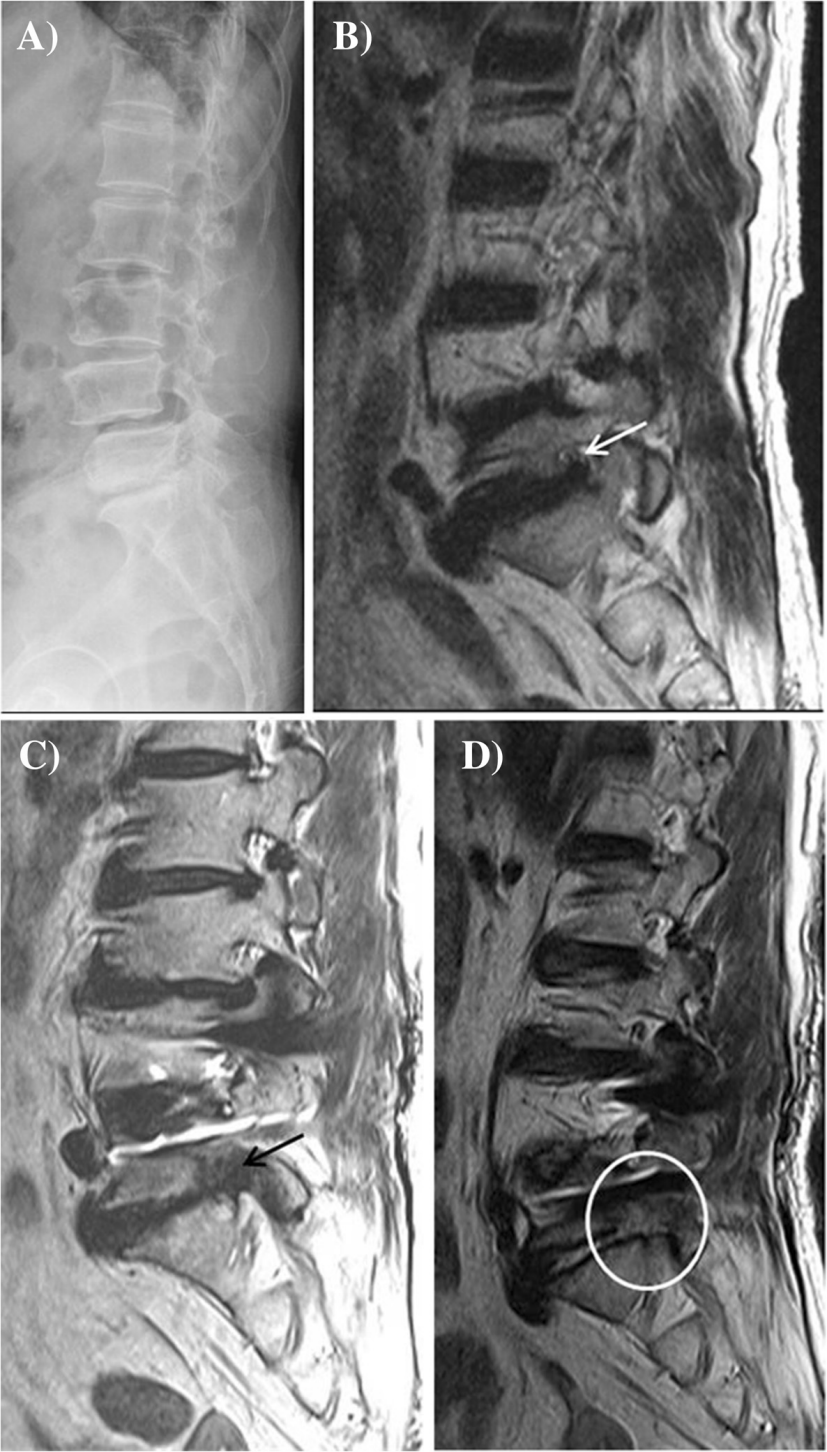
Imaging of degenerative spondylolisthesis in a 65-year-old woman. (A) Lateral radiography reveals degenerative spondylolisthesis at L4-5 and decreased disc height at the L5-S1 level. (B) T2-weighted right parasagittal magnetic resonance imaging (MRI) of the lumbar spine reveals spondylolisthesis at the L4-5 level and right foraminal stenosis (white arrow) of L5-S1. (C) MRI reveals aggravation of the foraminal stenosis (black arrow) of L5-S1 after anterior lumbar interbody fusion of L4-5 (10 years after surgery). (D) MRI reveals widening of the L5-S1 foramen (white circle) after decompression via the intermuscular approach at L5-S1.
